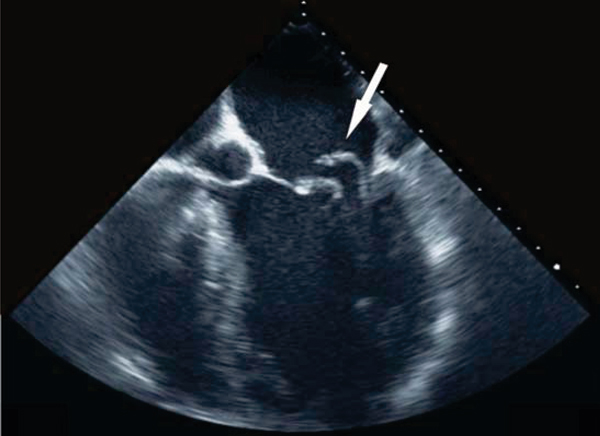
Transesophageal echocardiogram visualizing a flail posterior leaflet of the mitral valve.
